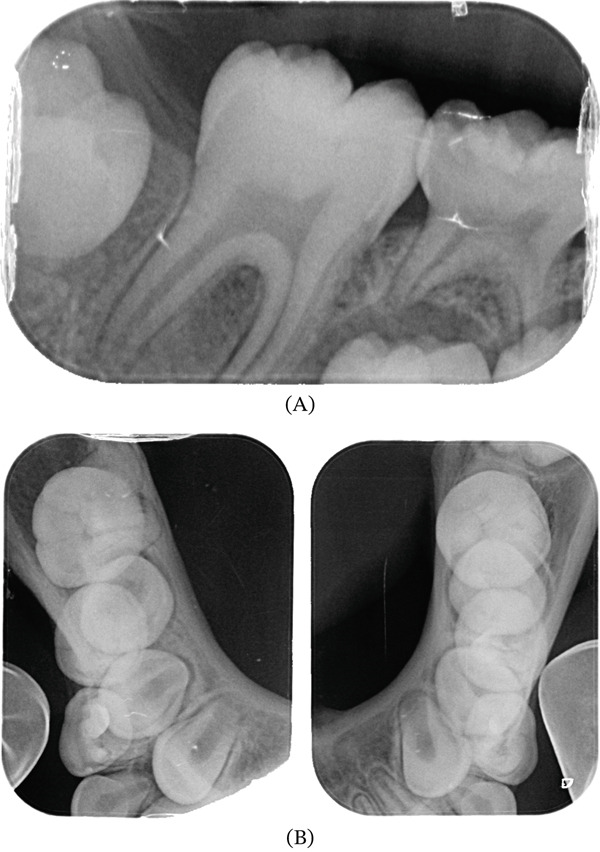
Radiography. (A) Periapical projection; and (B) occlusal projection.
